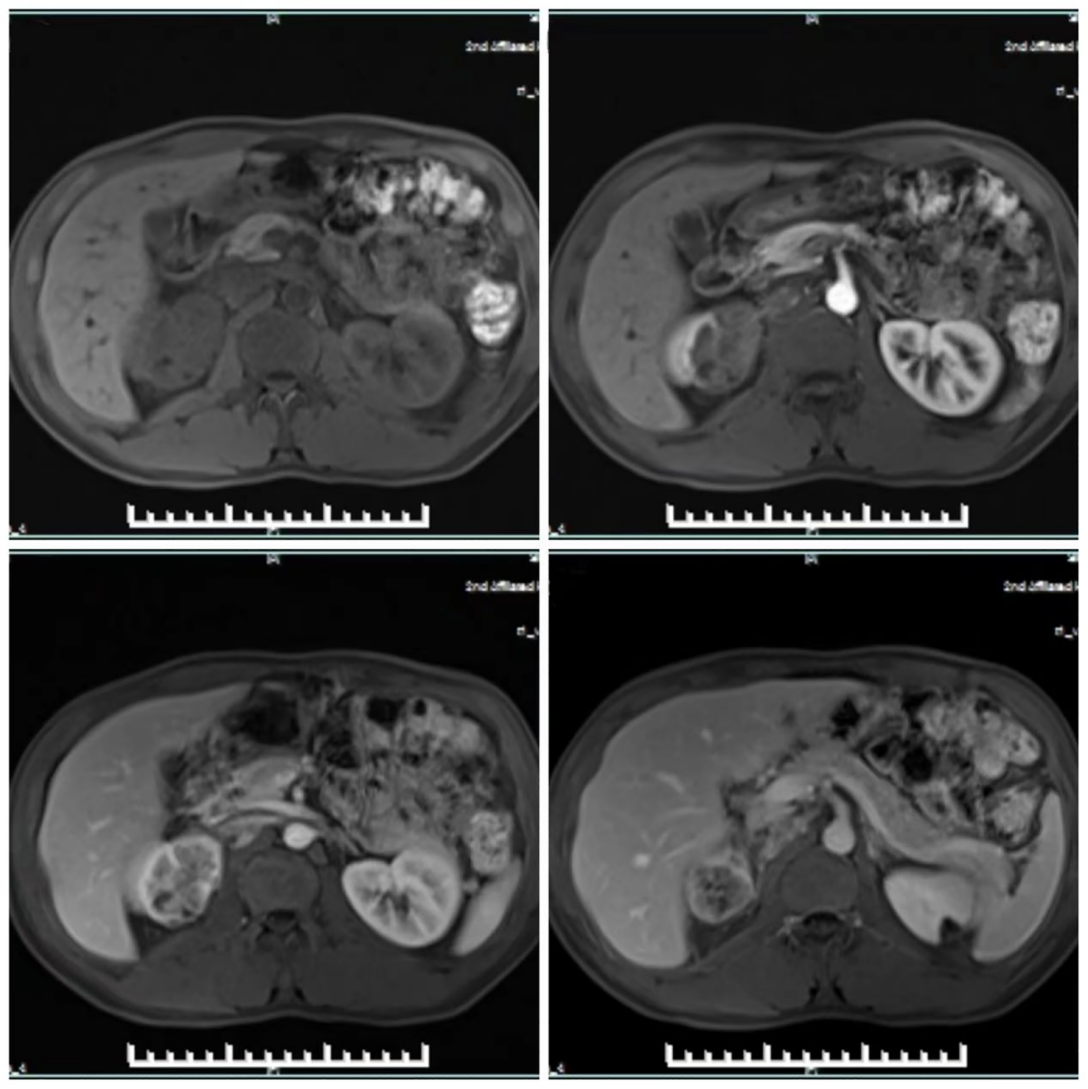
MRI showed heterogeneous enhancement in all phases of enhanced scanning, and multiple small cystic areas without enhancement were found in the tumor.
